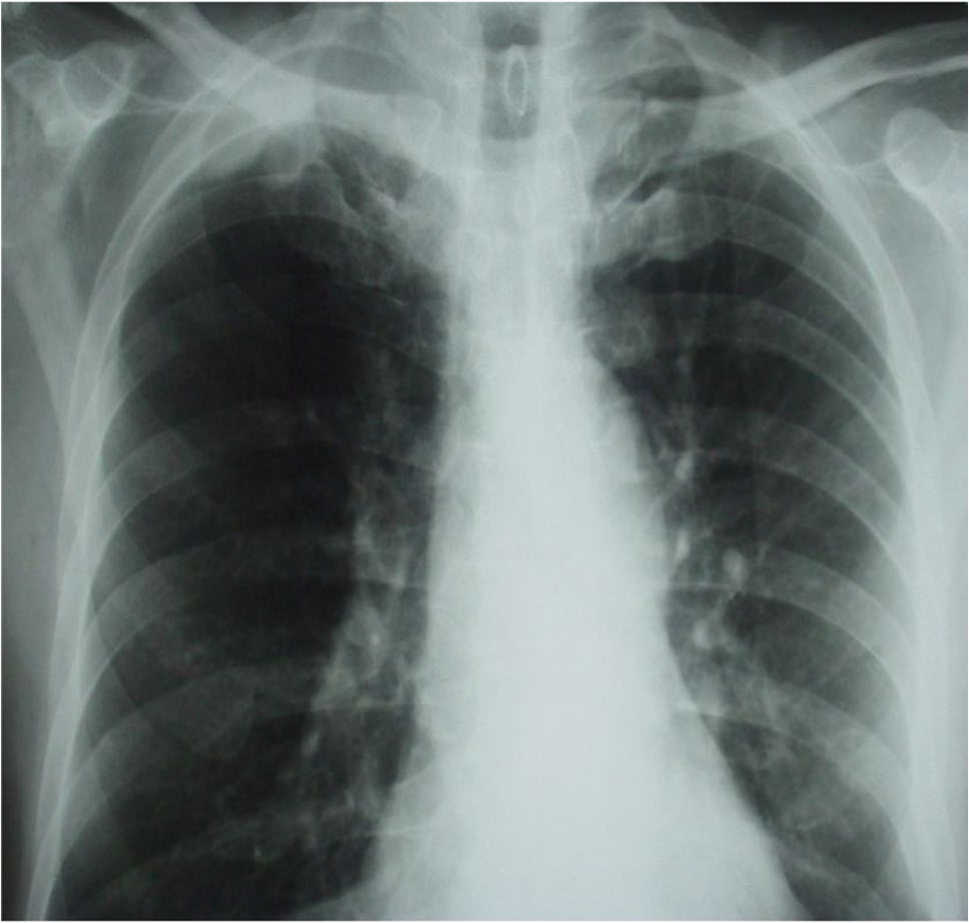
AP radiographs image of the chest
